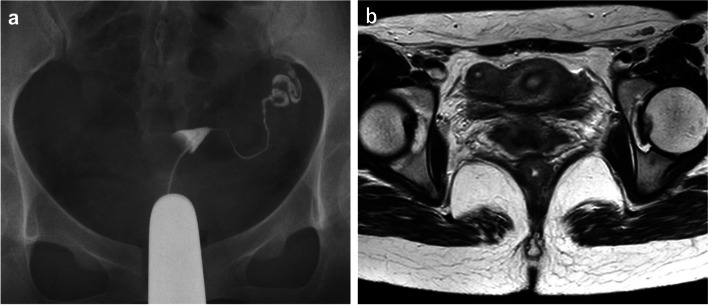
Diagnosis of unicornuate uterus with a non-communicating rudimentary horn. a Image of hysterosalpingography. b T2-weighted transverse magnetic resonance imaging
